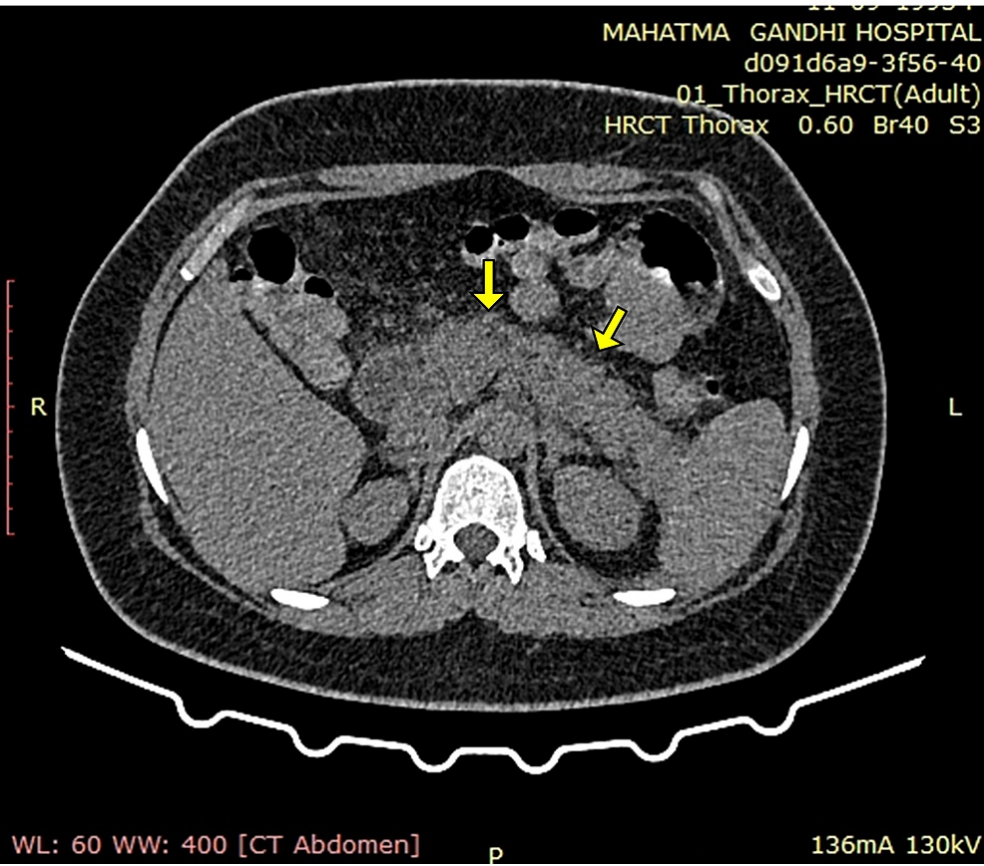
Non-contrast CT of the abdomen (axial section) showing diffusely bulky pancreas with no areas of calcification noted (solid yellow arrows).
